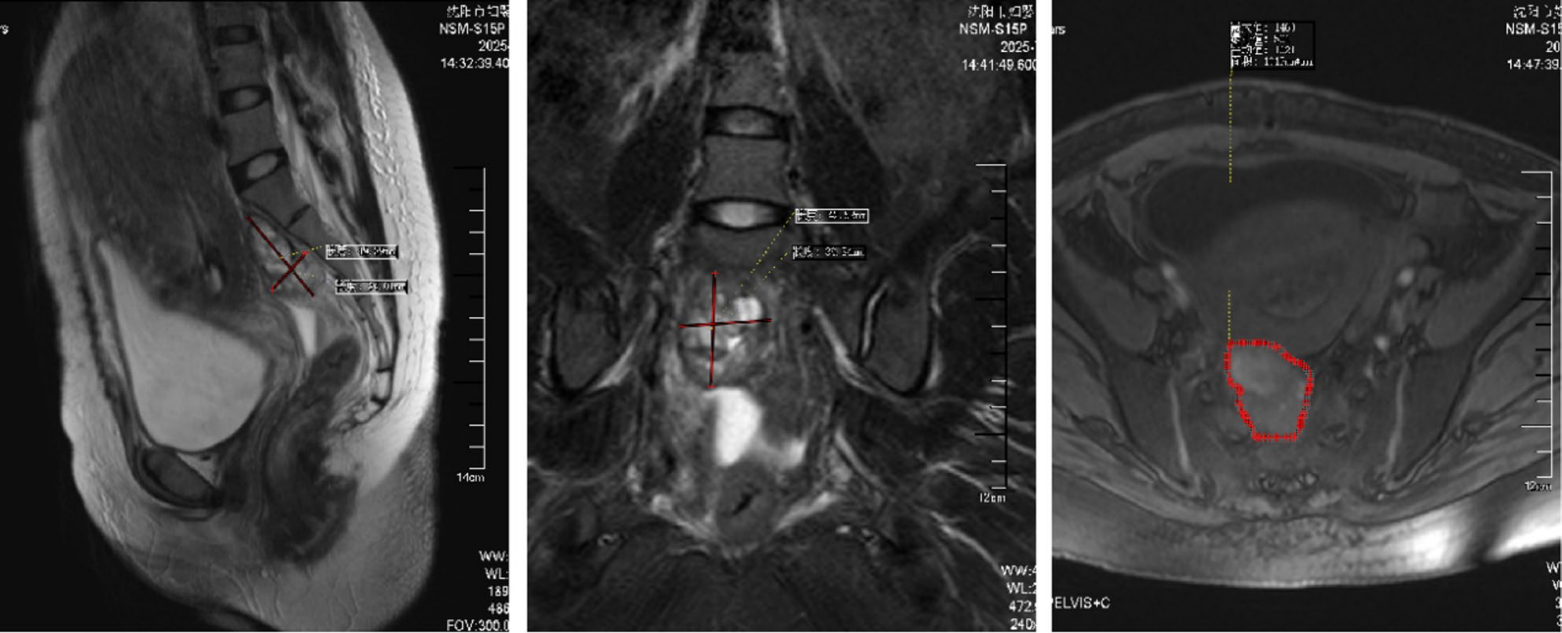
Postoperative MRI at 3 days after cesarean section. A mixed-signal mass (50 mm×26 mm×37 mm) posterior to the lower uterus, consistent with a partially organized hematoma
